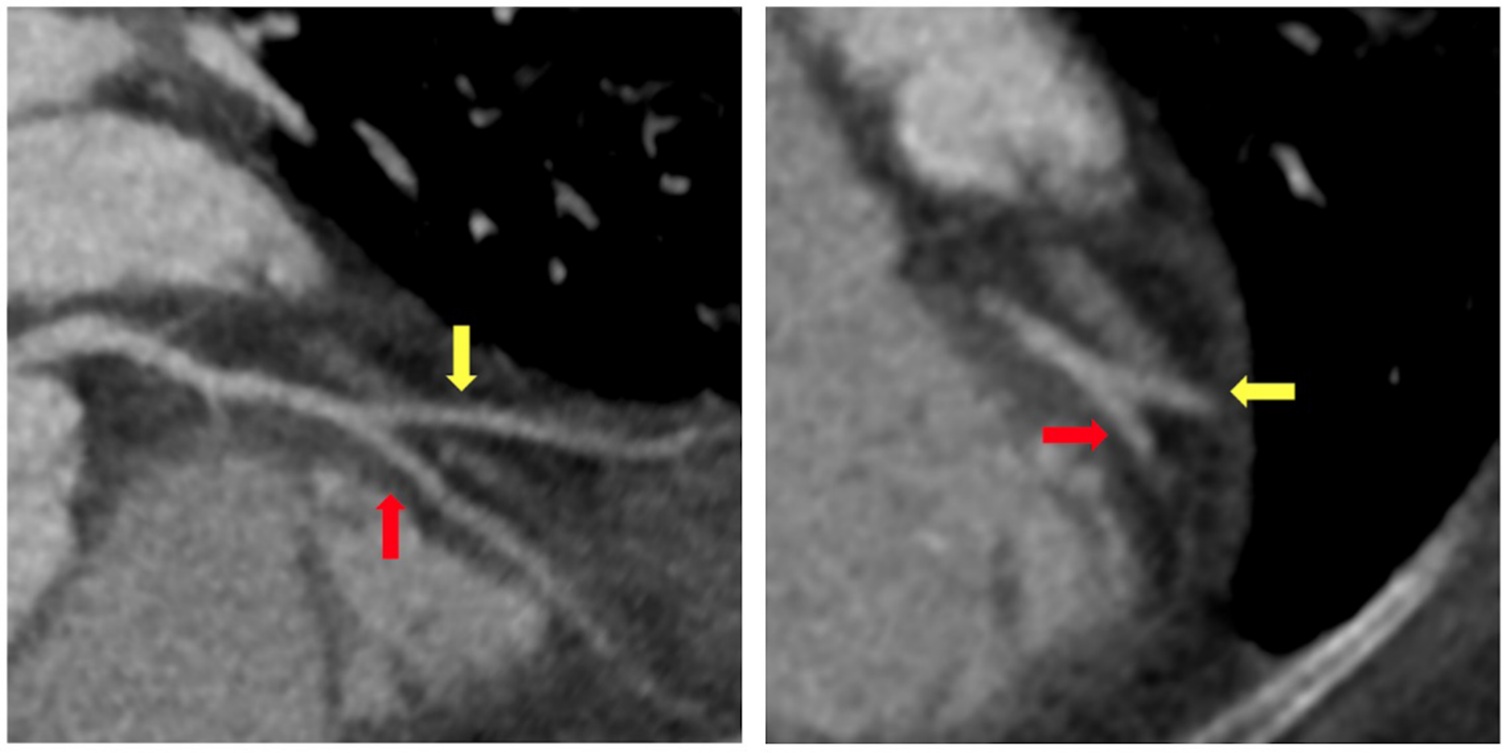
Coronary computed tomography angiography. Coronary computed tomography angiography performed 6 weeks prior to index hospitalization for acute coronary syndrome demonstrated minimal coronary atherosclerosis at the bifurcation of the mid-left anterior descending artery (red arrow) and secondary diagonal artery (yellow arrow).
